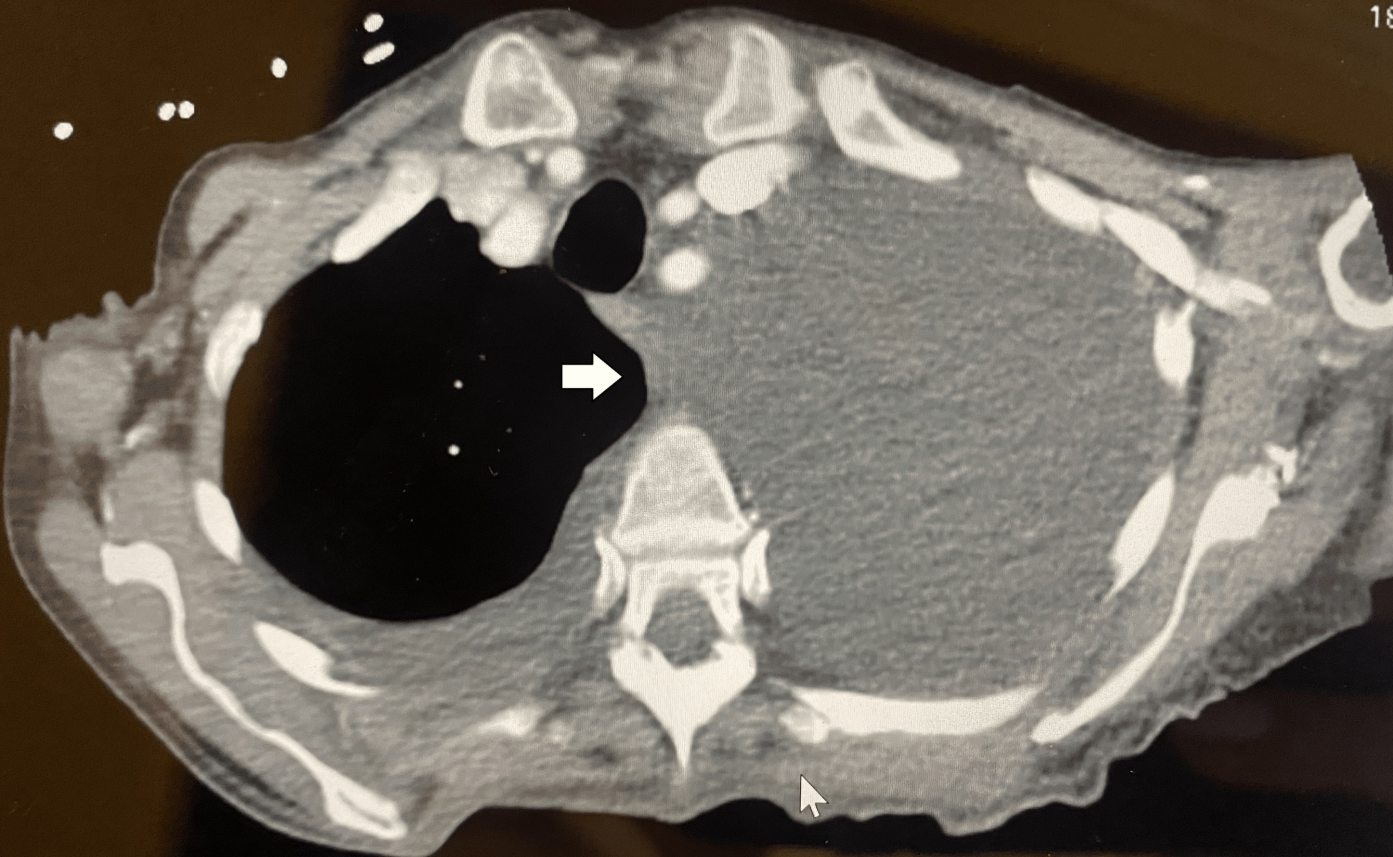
CT of the thorax with contrast axial view with complete opacification of the left lung before thoracentesis.
